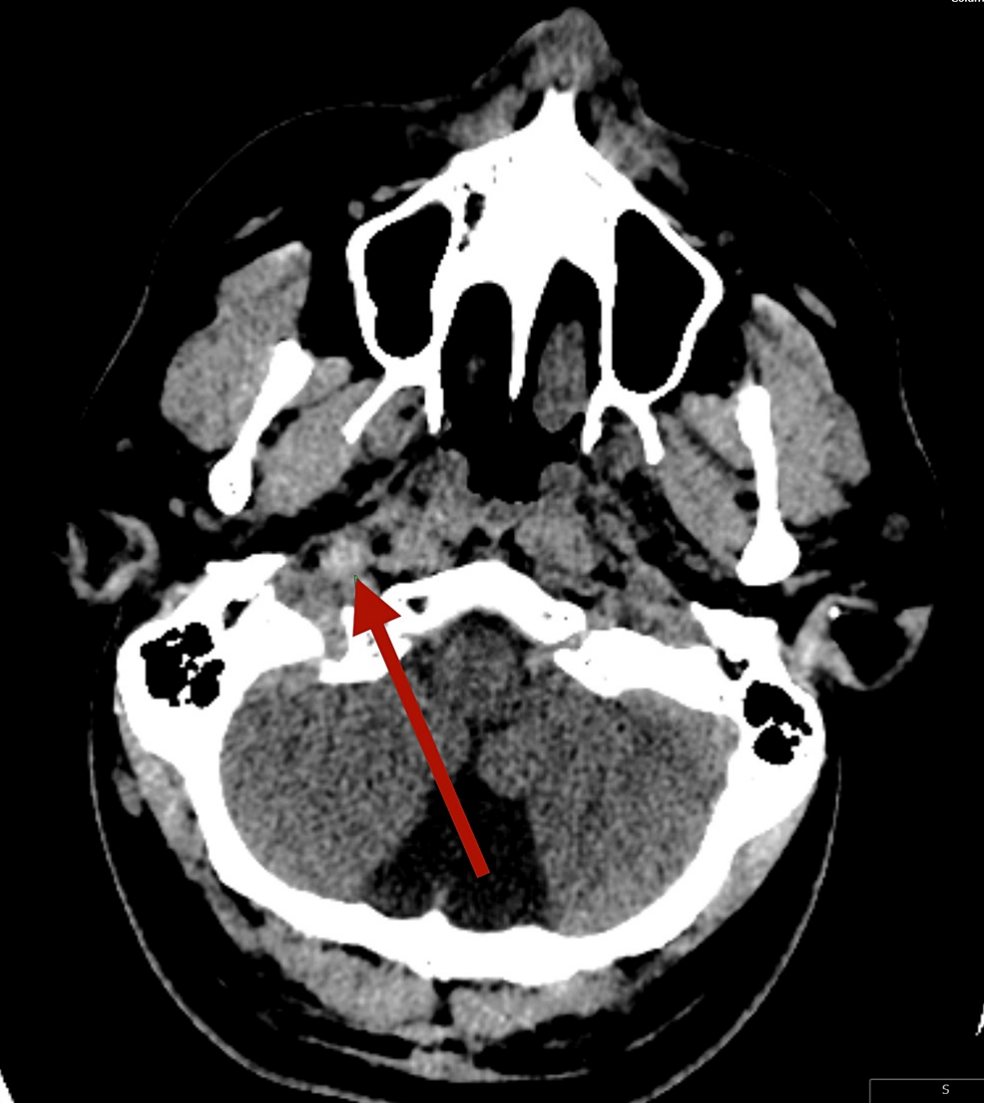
Non-contrast CT scan showing hyperdensity in right ICA at skull base ICA: internal carotid artery
